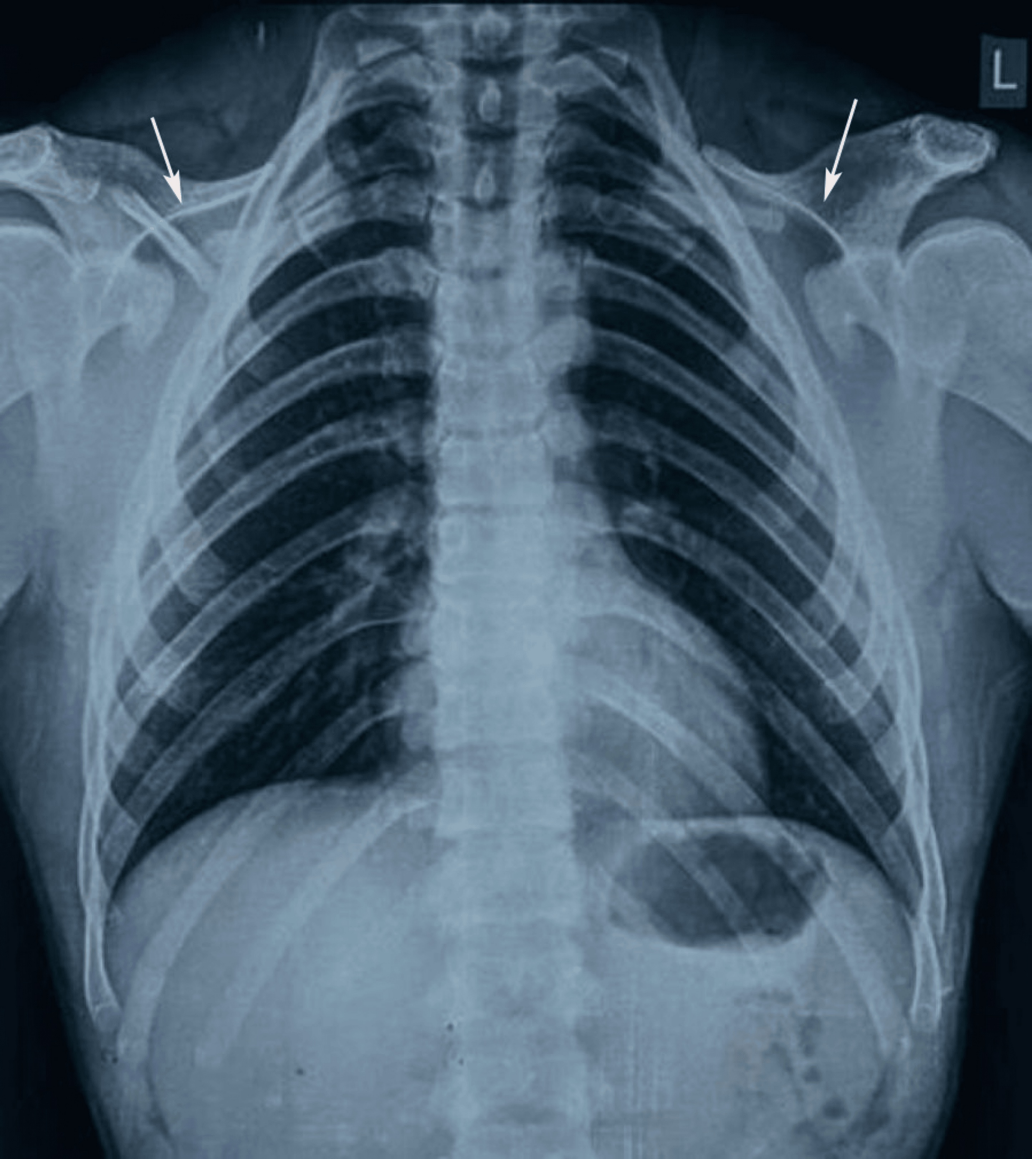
Chest X-ray showing bilateral hypoplastic clavicles (white arrows), a characteristic feature of cleidocranial dysplasia
